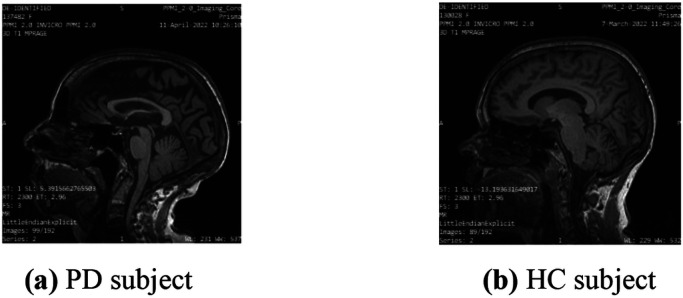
Original MRI brain images of T1,T2-weighted dataset (a) PD subject (b) HC subject.
